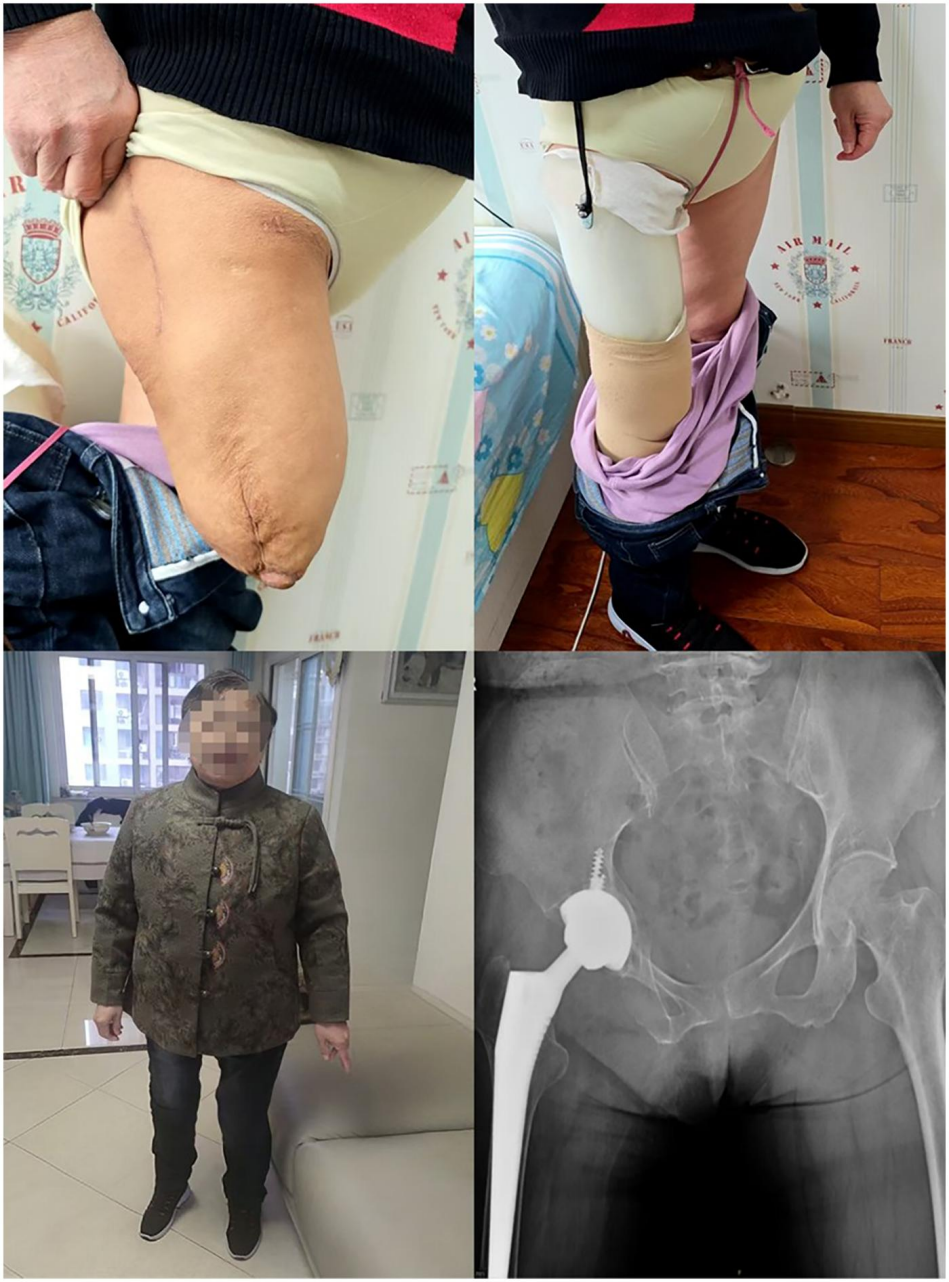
Postoperative radiographs and clinical pictures with prosthesis at 1 year-follow-up.
